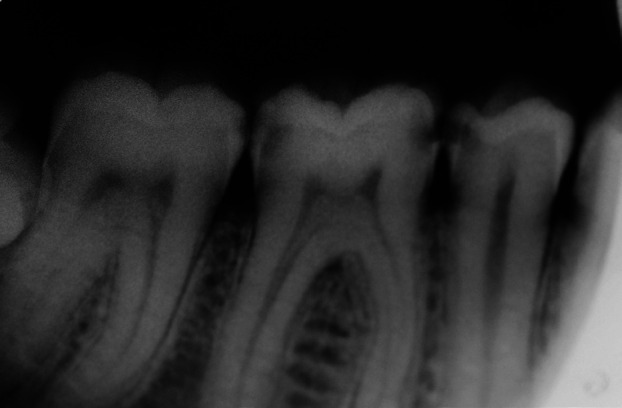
Radiographic image of the fourth quadrant.
